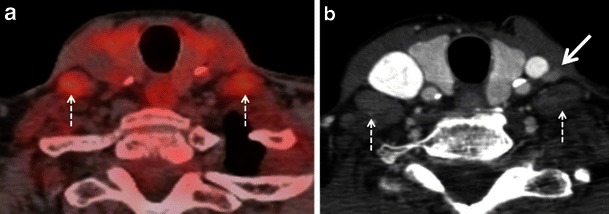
a Axial PET/CT image shows no evidence of abnormal FDG uptake or metastatic cervical adenopathy in the neck. Physiological mild FDG uptake is seen in the thyroid gland, oesophagus and in the scalenus muscles (*dashed arrows*). This patient was a follow-up case of a SCC of the base of the tongue. b Corresponding CECT image (1 mm slice) detects a 5 x 7 mm sized enhancing node at left level IV (*white arrow*). This node did not show an increased FDG uptake on the PET acquisition of the PET/CT (slice thickness of PET acquisition was 5 mm). US FNAC revealed metastatic lymphadenopathy. Neck dissection confirmed metastasis from HNSCC. Dashed arrows in b point at normal anterior scalenus muscles
